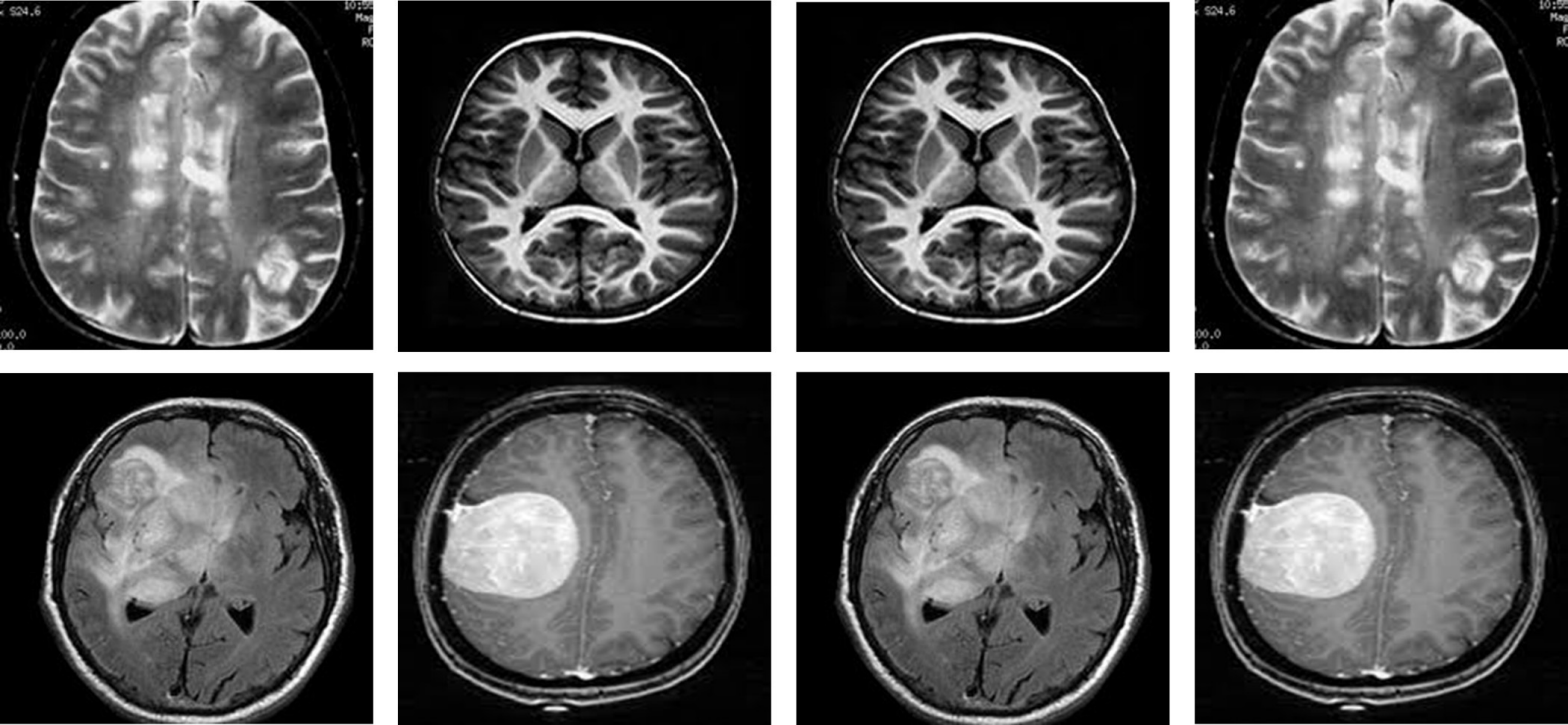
Samples of BTD-MRI dataset, upper row: Non-tumor, lower row: Tumorous.
